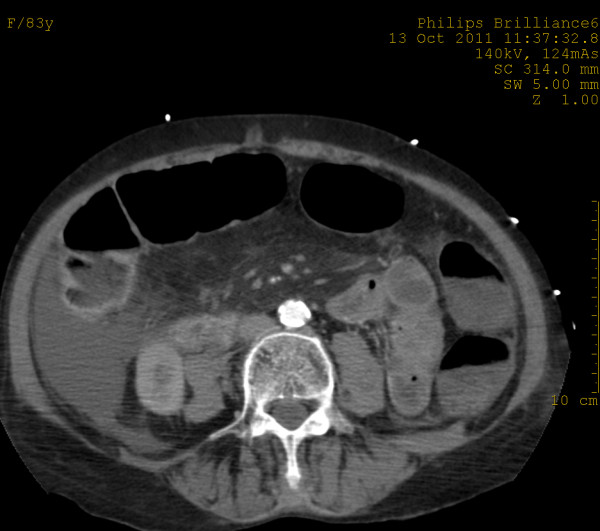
Axial CT image at venous phase shows intraperitoneal fluid and pericaecal fat stranding.
